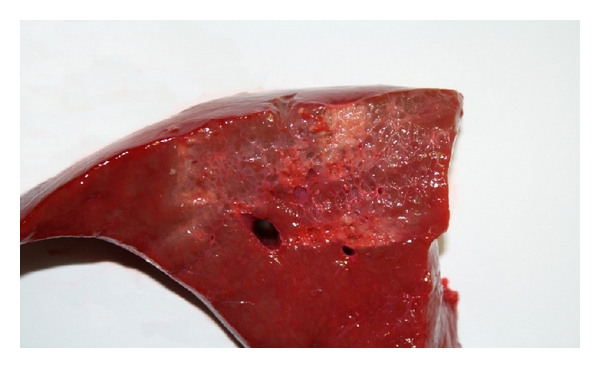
Liver tissue after 15-min ultrasonic coagulation.
